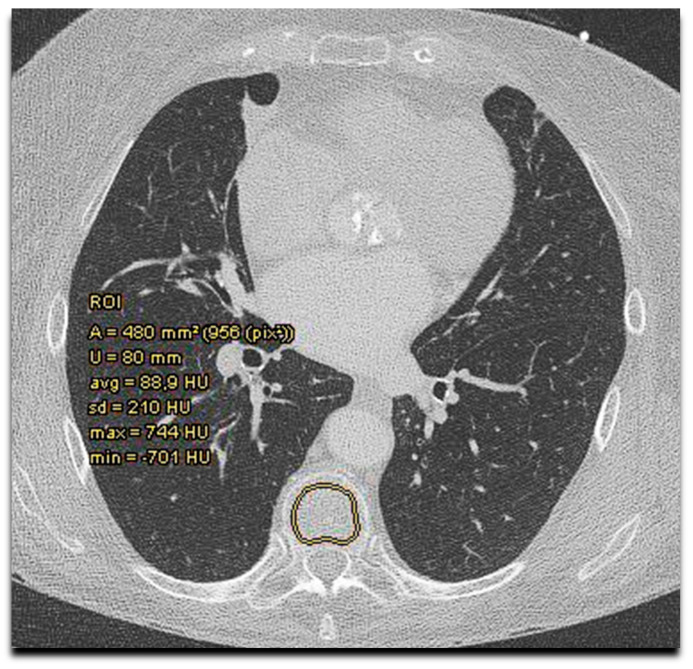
Bone mineral density assessment by Hounsfield units measurement on TAVR-CT scan. Bone mineral density (Hounsfield units) measurement in sternum and thoracal vertebra with determination of area (A), circumference (U), average (avg) density, standard deviation (SD), and maximal (max) and minimal (min) density in the region of interest (ROI).
